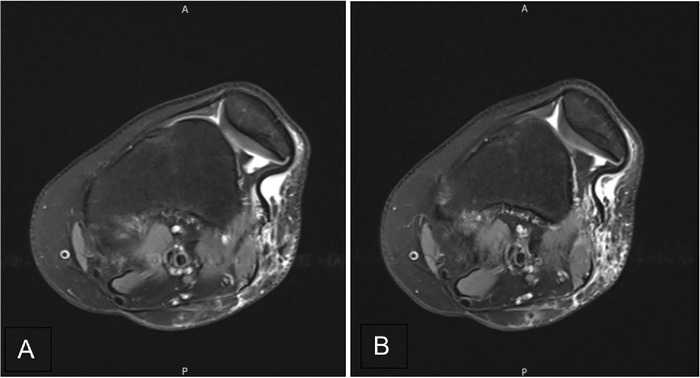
(A, B) Preoperative magnetic resonance imaging of the left knee with cartilage-specific sequences shows severe lateral patellar subluxation but minimal articular cartilage damage.
